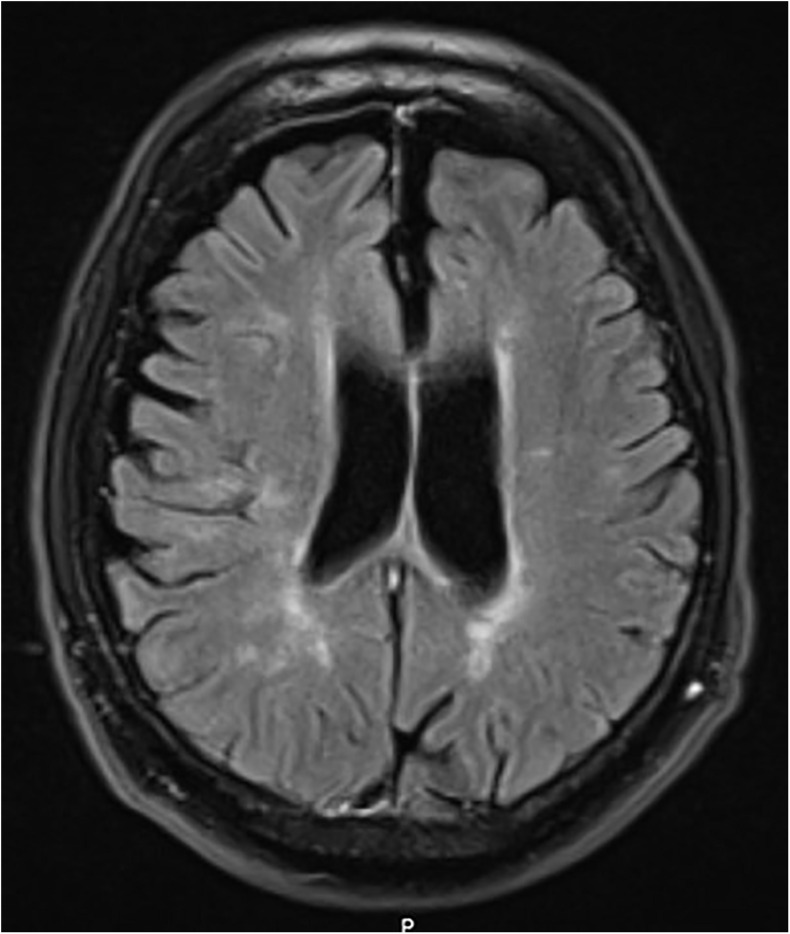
Case 3, LRRK2 mutation carrier, severe MS. MRI at age 50 with white matter lesions and some central and cortical brain atrophy.
